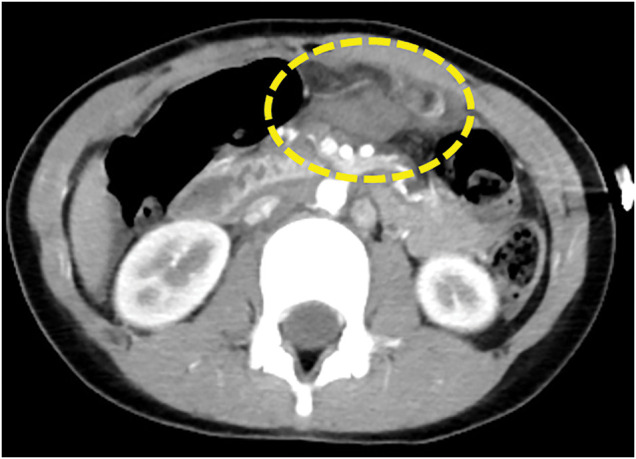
Contrast-enhanced CT 2 h after injury. Duodenal injury was suspected in the area marked by the yellow dotted line.
